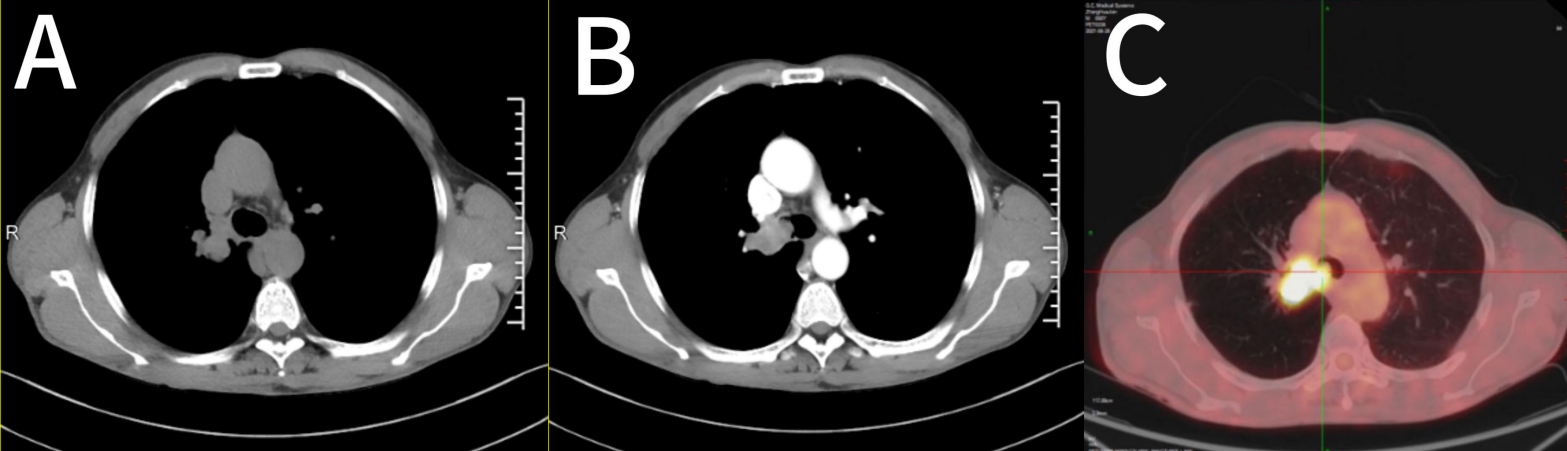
Imaging features of the case. (A, B) Chest CT showed a right main bronchial mass, approximately 27×32 mm. (C) PET-CT showed a radioactive catheter uptake mass in the lumen of the main bronchial tube of the right lung. CT, computed tomography; PET, positron emission tomography.
